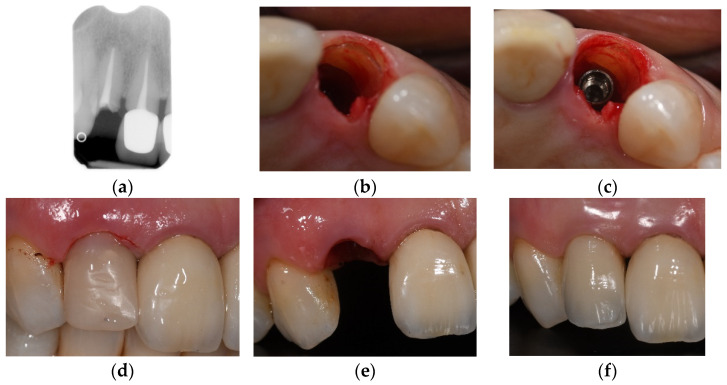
Case of socket shield technique and immediate implant placement. (a) the preoperative X-ray shows the absence of apical and/or periodontal infection (b,c) the root shield is preserved buccally and slightly more apical from the gingival margin (d) temporary crown in acrylic resin- no functional loading (e) emergence profile 3 months after the procedure (f) one year follow up.
